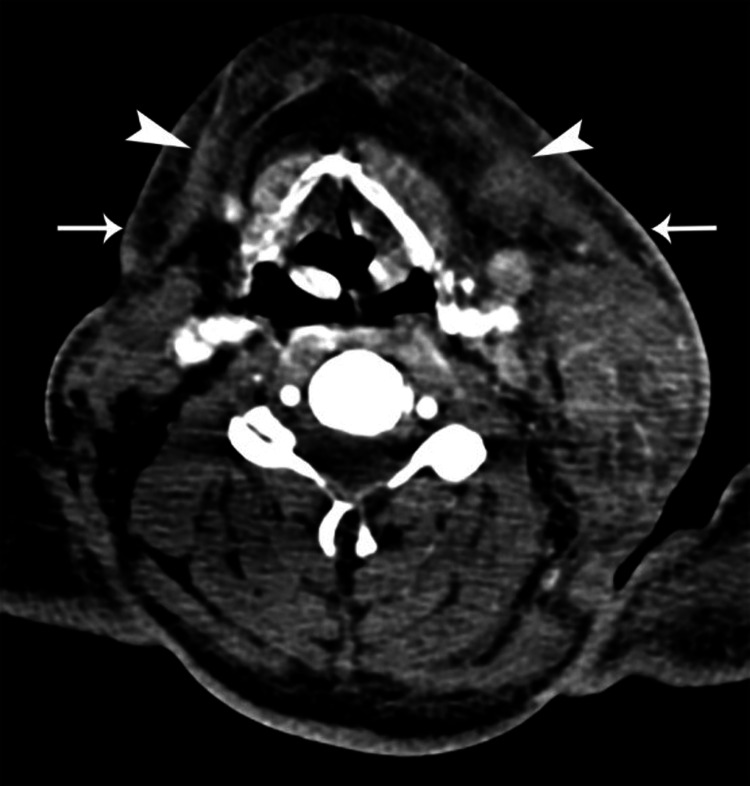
CT scan of the neck showing diffuse thickening of cutis, subcutis (arrows), and platysma (arrowheads) with fat stranding.
